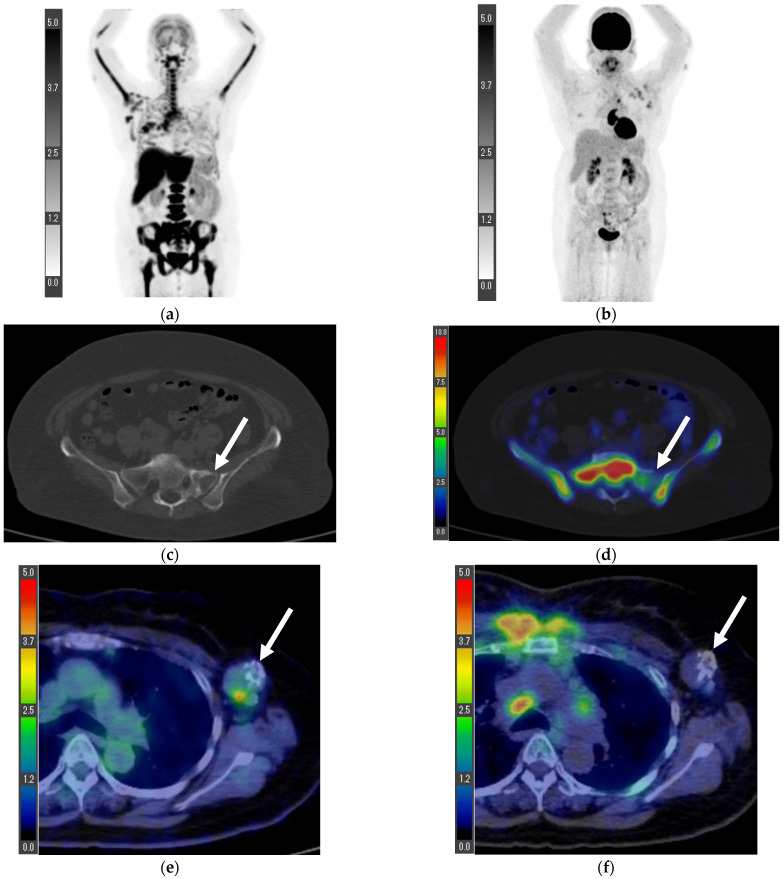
Maximum intensity projection (MIP) of ^18^F-FLT PET (a) and ^18^F-FDG PET 10 months prior (b). ^18^F-FLT accumulation within an osteolytic lesion ((c), arrow) on the left side of the sacrum was lower than that in the physiological bone marrow ((d), arrow). In addition, left axillary lymph node accumulation with coarse calcification was found on ^18^F-FDG PET/CT ((e), arrowhead); however, accumulation on ^18^F-FLT PET/CT did not increase ((f), arrowhead), suggesting inflammation. Patient 8 (Figure 6).
